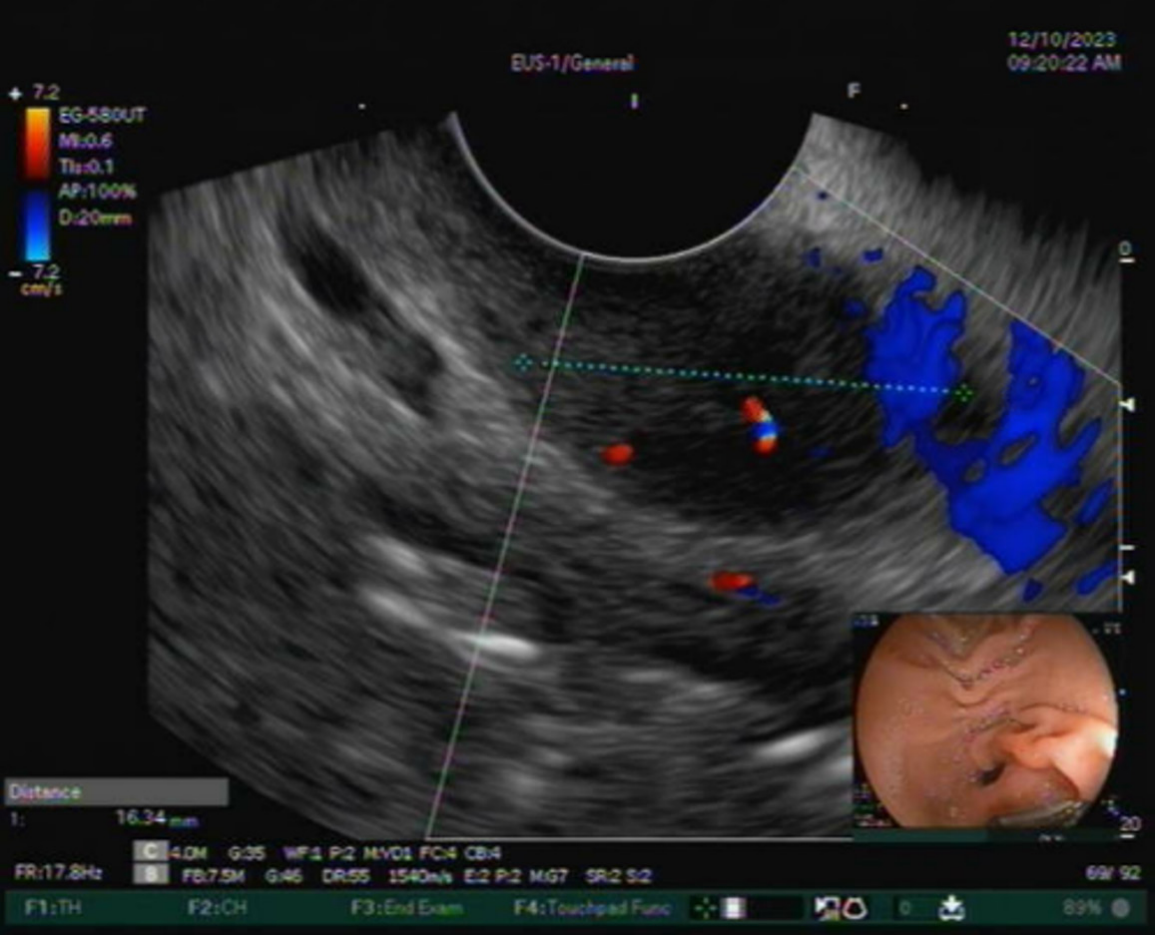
Endoscopic ultrasound showing a well‐defined subepithelial lesion of 16 mm diameter with internal vascularity located at the duodenal bulb.
